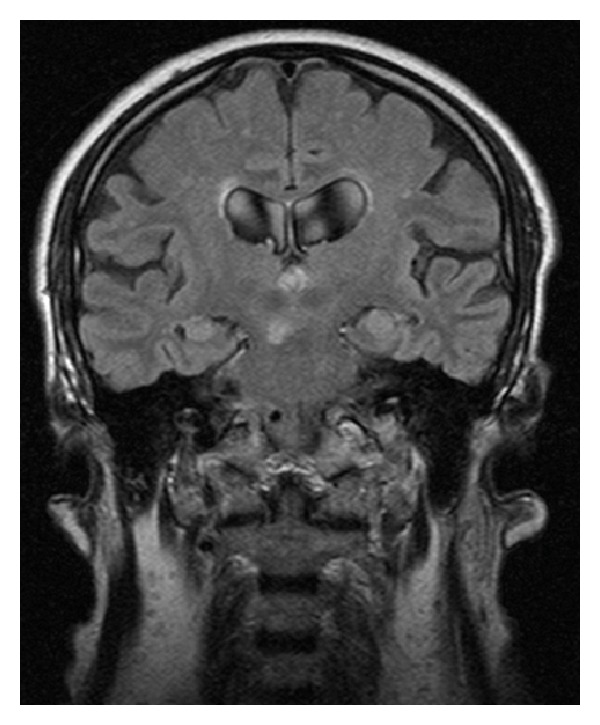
MRI Flair—ischemic lesion at the cerebral trunk evolving the right median portion.
